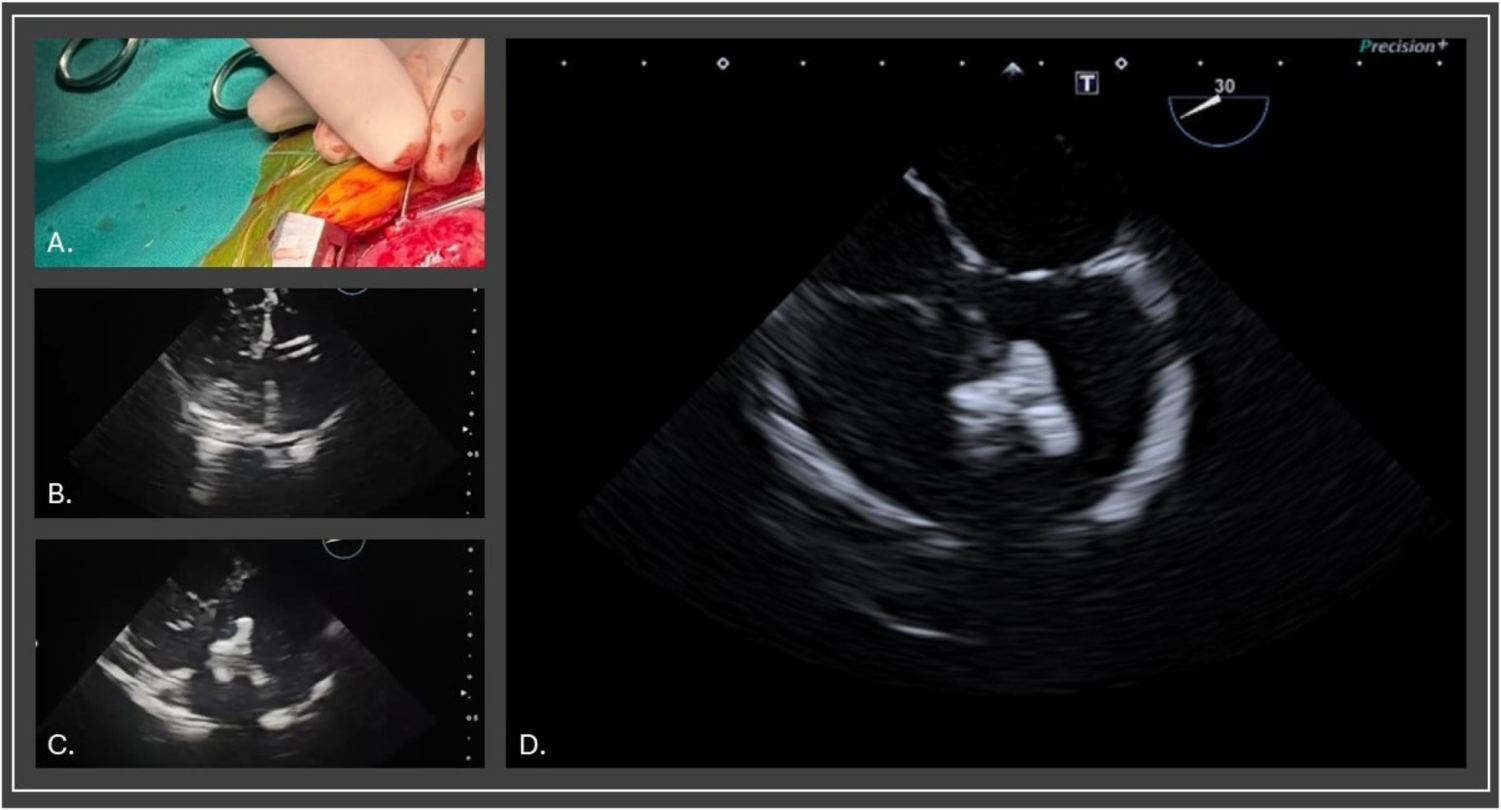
A right ventricular free wall puncture (A) allowed placement of a 6-F short sheath across the VSD into the left ventricle (B) transesophageal echocardiography revealed an amplatzer ductal occluder (ADO) 1 connected to the delivery cable (C) position of the ADO1 after deployment (D).
